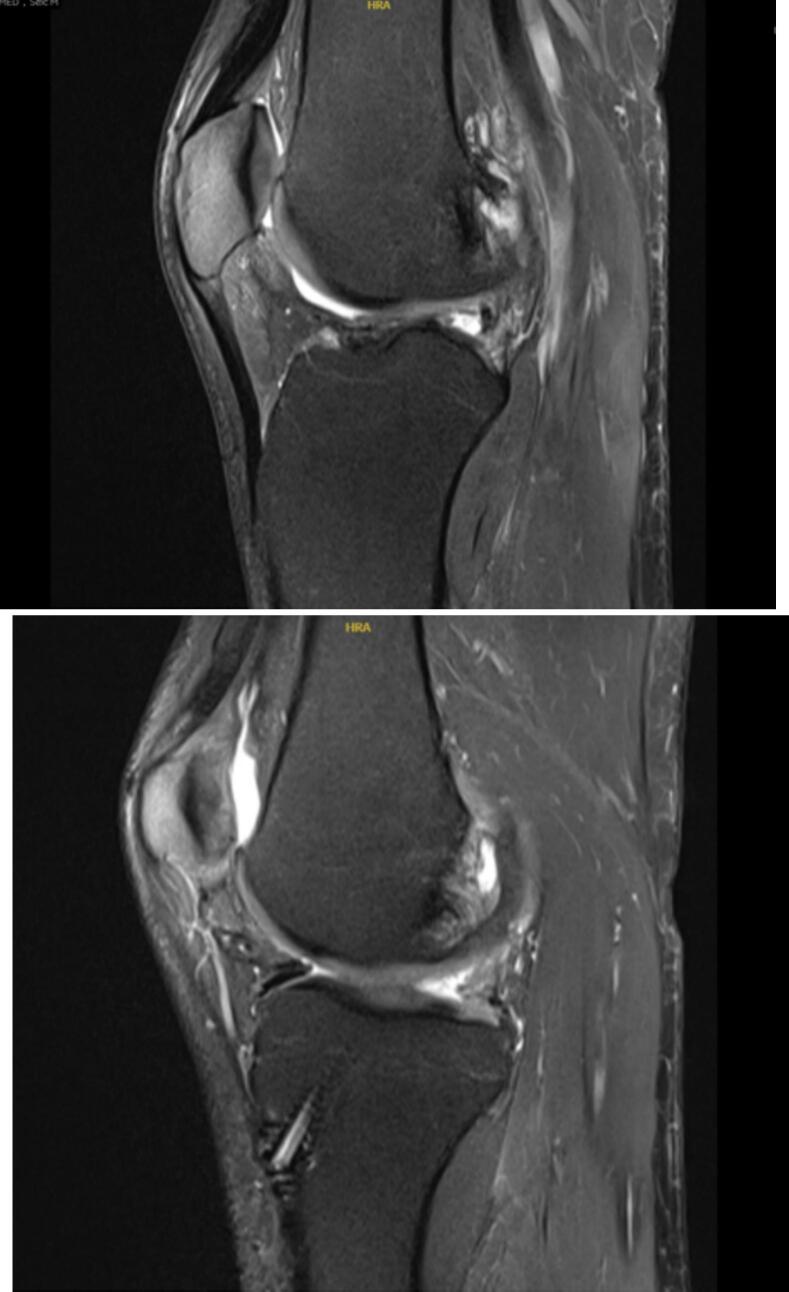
T2 magnetic resonance imaging sagittal cuts showing increased signals at the posterior roots of lateral (a) and medial (b) meniscus.
